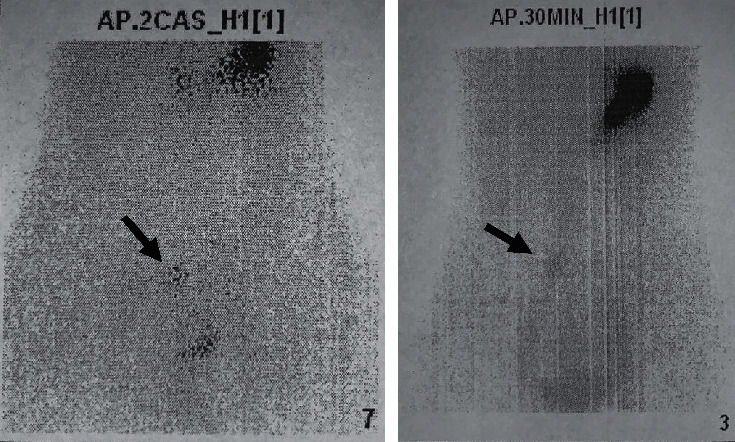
Abdominal scintigraphy with 99 m Tc pertechnetate (Meckel scan) showed abnormal accumulation of the radioisotope indicating the presence of ectopic gastric mucosa. The black arrows point to the areas of abnormal accumulation.
